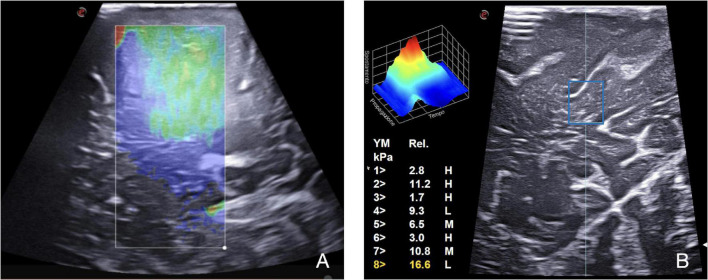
SE and SWE. Elastosonography provides information regarding the tumor and surrounding parenchyma mechanical properties through qualitative and quantitative evaluation. (A) On the left, SE in a case of metastasis: a mechanical force is applied to measure lesion stiffness, which is expressed on a color scale for qualitative assessment. (B) On the right, SWE, using the QElaxto algorithm, provides a quantitative assessment of stiffness of a specific ROI inside the lesion.
